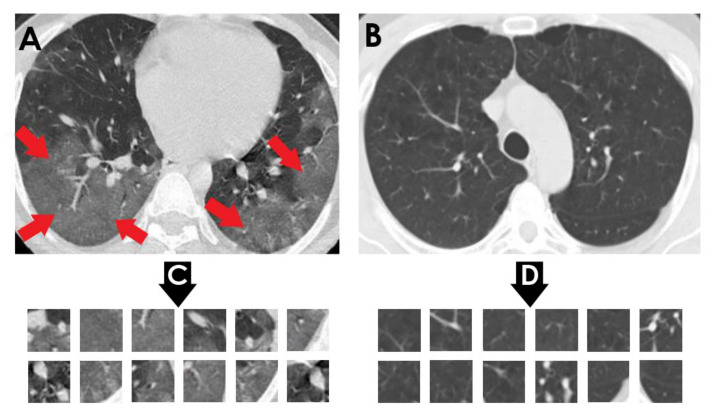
(A) Shows a CT of the lungs of COVID-19 (+) patient, in which a ground-glass opacity is visible in the lower lobes (red arrows). (B) Represents a CT of the lungs of COVID-19 (−) patient, in which there are no abnormalities. (C) Depicts infected patch samples. (D) Reflects non-infected patch samples. SARS-CoV-2 CT-scan dataset [42] is the source for these images.
